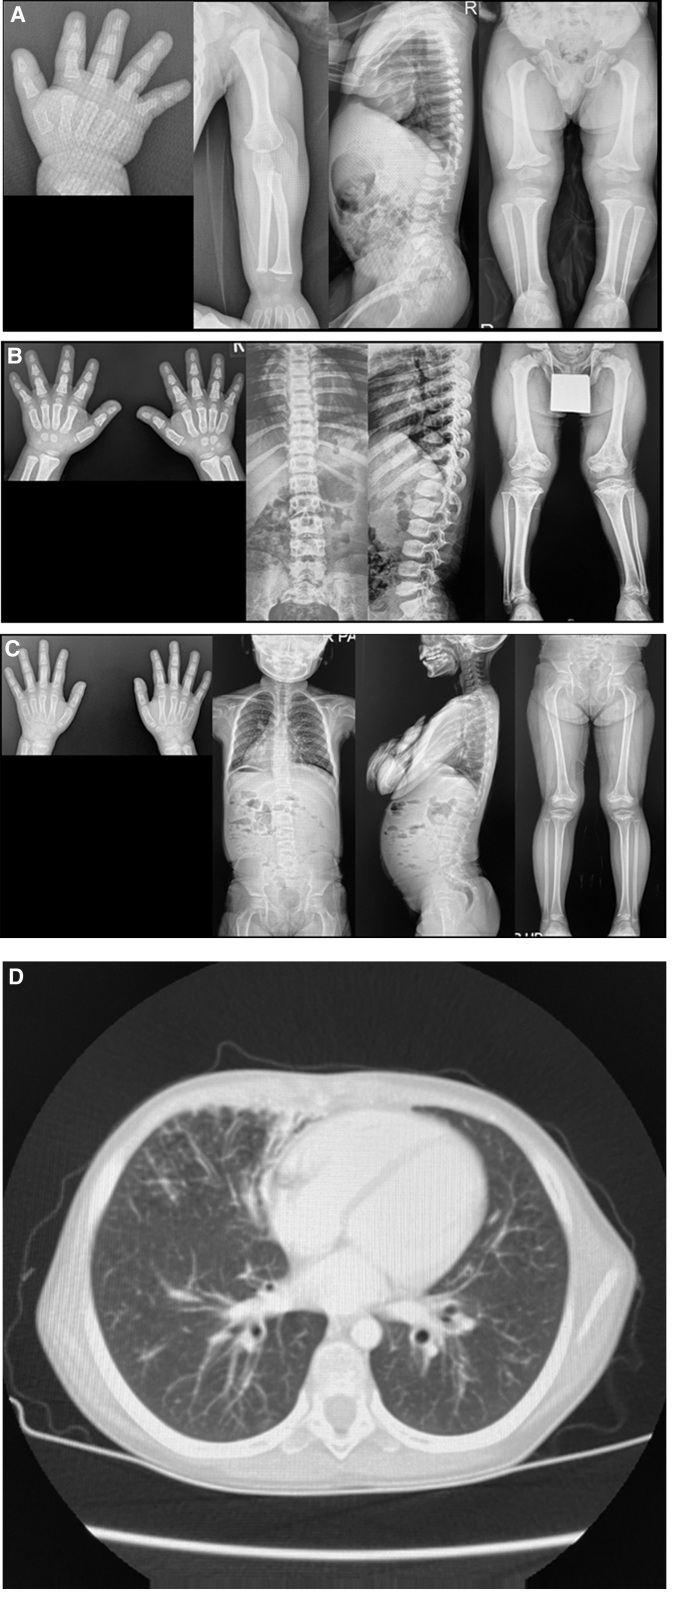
Skeletal survey of both patients and chest computed tomography (CT) of case 2. (A) Radiographs of the patient in case 1, aged 13 mo; both hands and distal forearms show metaphyseal irregularities and sclerosis of the distal radius and ulnae and mildly cone-shaped epiphyzes of the phalanges. They also show kyphosis and genu varum. (B) Radiographs of the patient in case 1, aged 8 yr and 2 mo. (C) Radiographs of the patient in case 2, aged 8 yr and 9 mo; both knees and hips show metaphyseal irregularities and sclerosis. (D) Chest CT of the patient in case 2 aged 10 yr and 1 mo.
